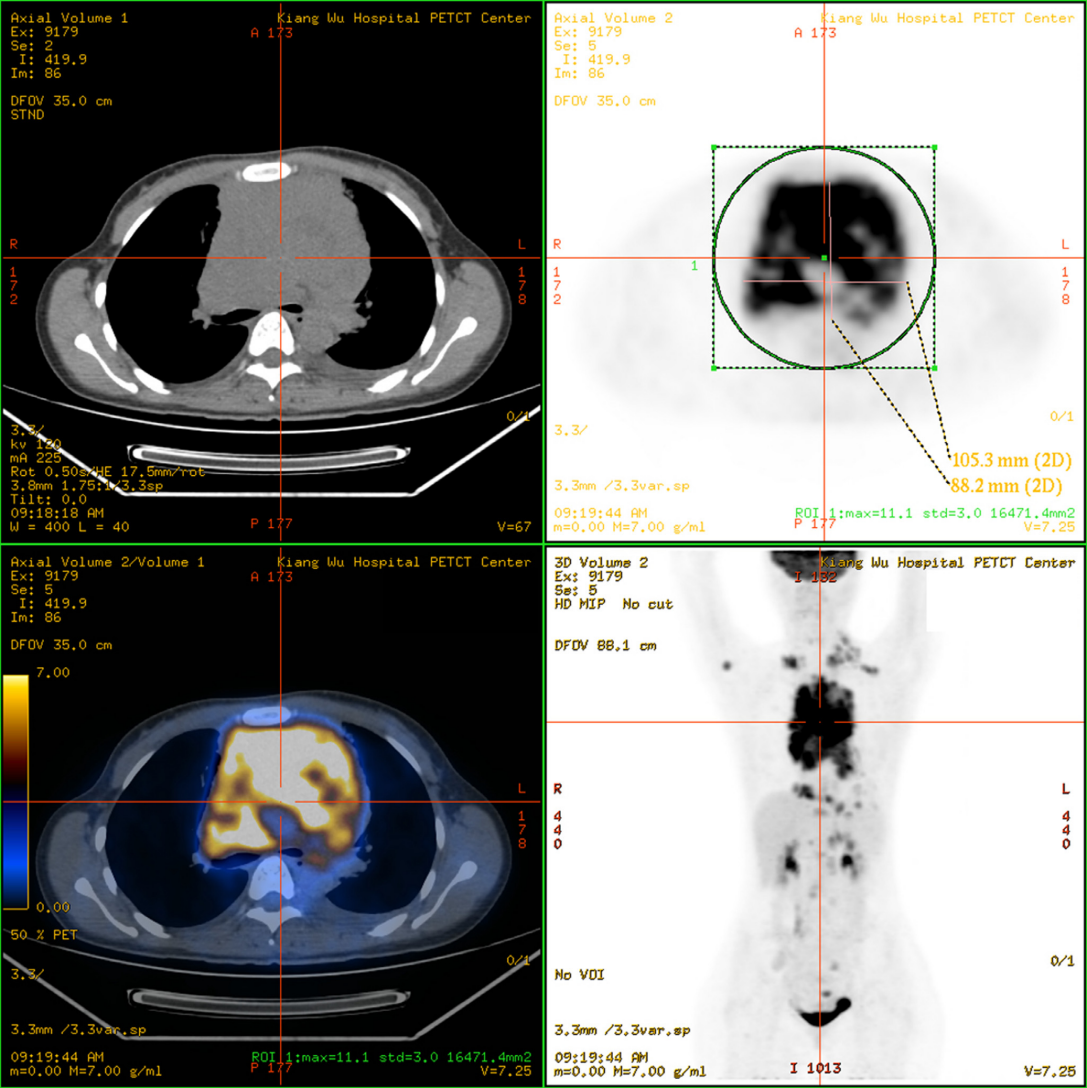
A 31-year-old female suffered from PMBCL with a baseline ^18^F-FDG PET/CT scan. An intensely hypermetabolic mass in the mediastinum accompanying multiple active lymph nodes, active lung lesions and bony foci.
